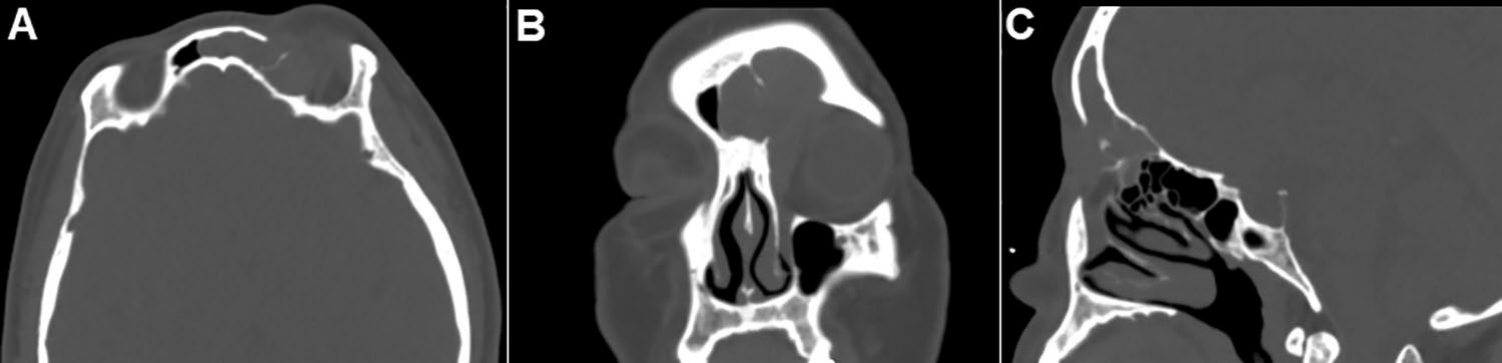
(A) Axial, (B) coronal and (C) sagittal paranasal sinus CT images of a patient with recurrent frontal sinus inverted papilloma who suffered by persistent headache
